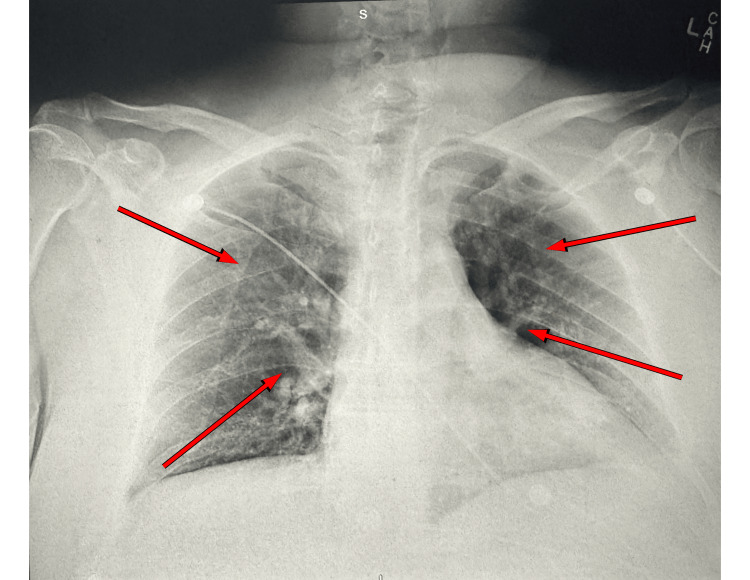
Chest radiograph obtained during follow-up in January 2026 demonstrating mild diffuse bilateral increased interstitial markings, highlighted by arrows. No focal consolidation or pleural effusion was identified.
